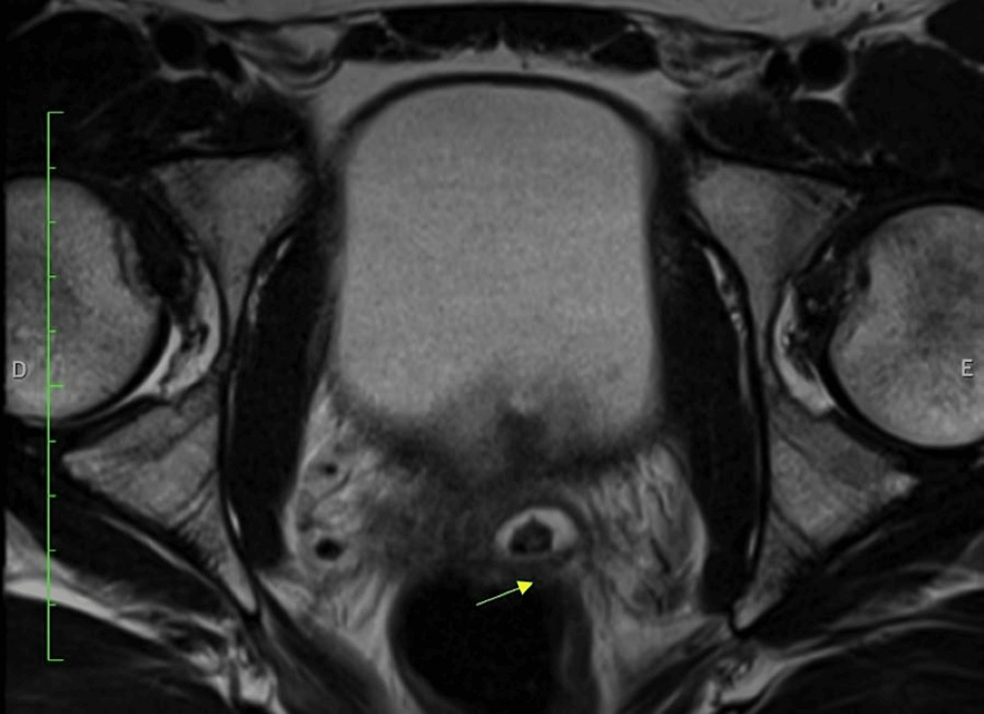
T2 MRI axial view. The arrow shows the left seminal vesicle with the calculi inside. MRI: magnetic resonance imaging
